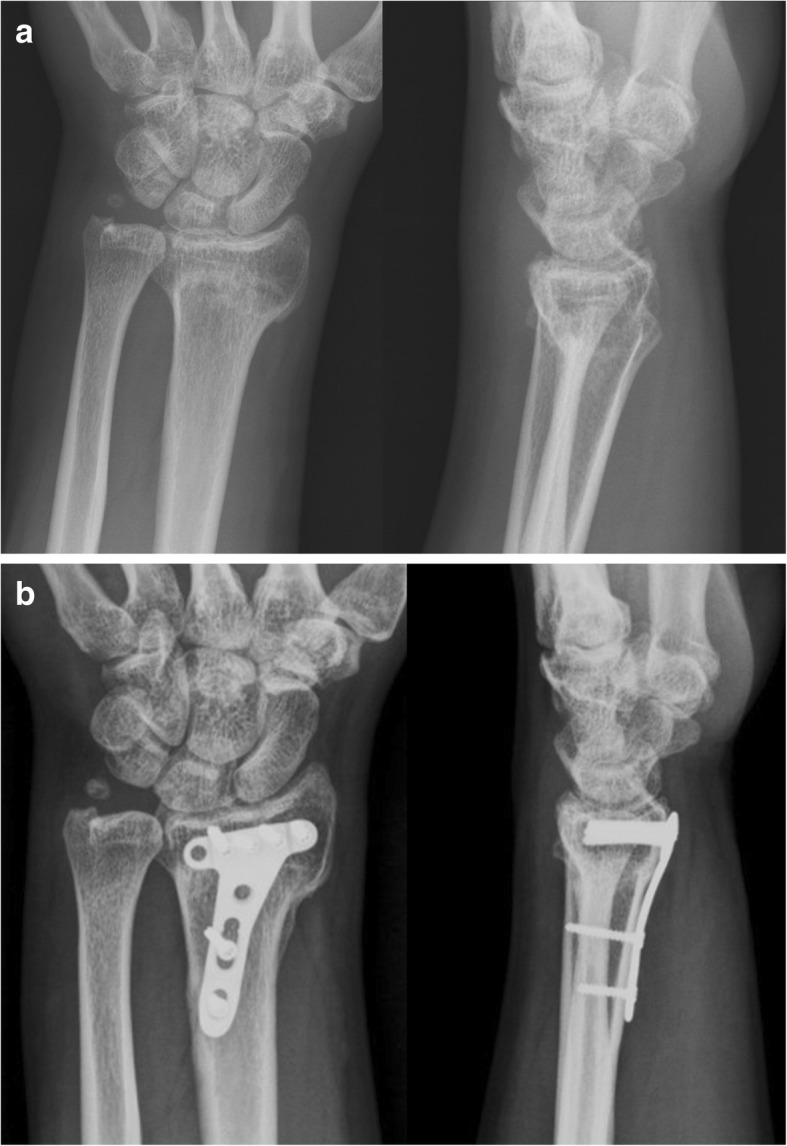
A 39-year-old male patient with a distal radius fracture of 28 weeks. a Preoperative radiographs showing malunion, posteroanterior, and lateral projections. b Postoperative radiographs of volar locking plate 2 years after surgery showing posteroanterior and lateral projections
